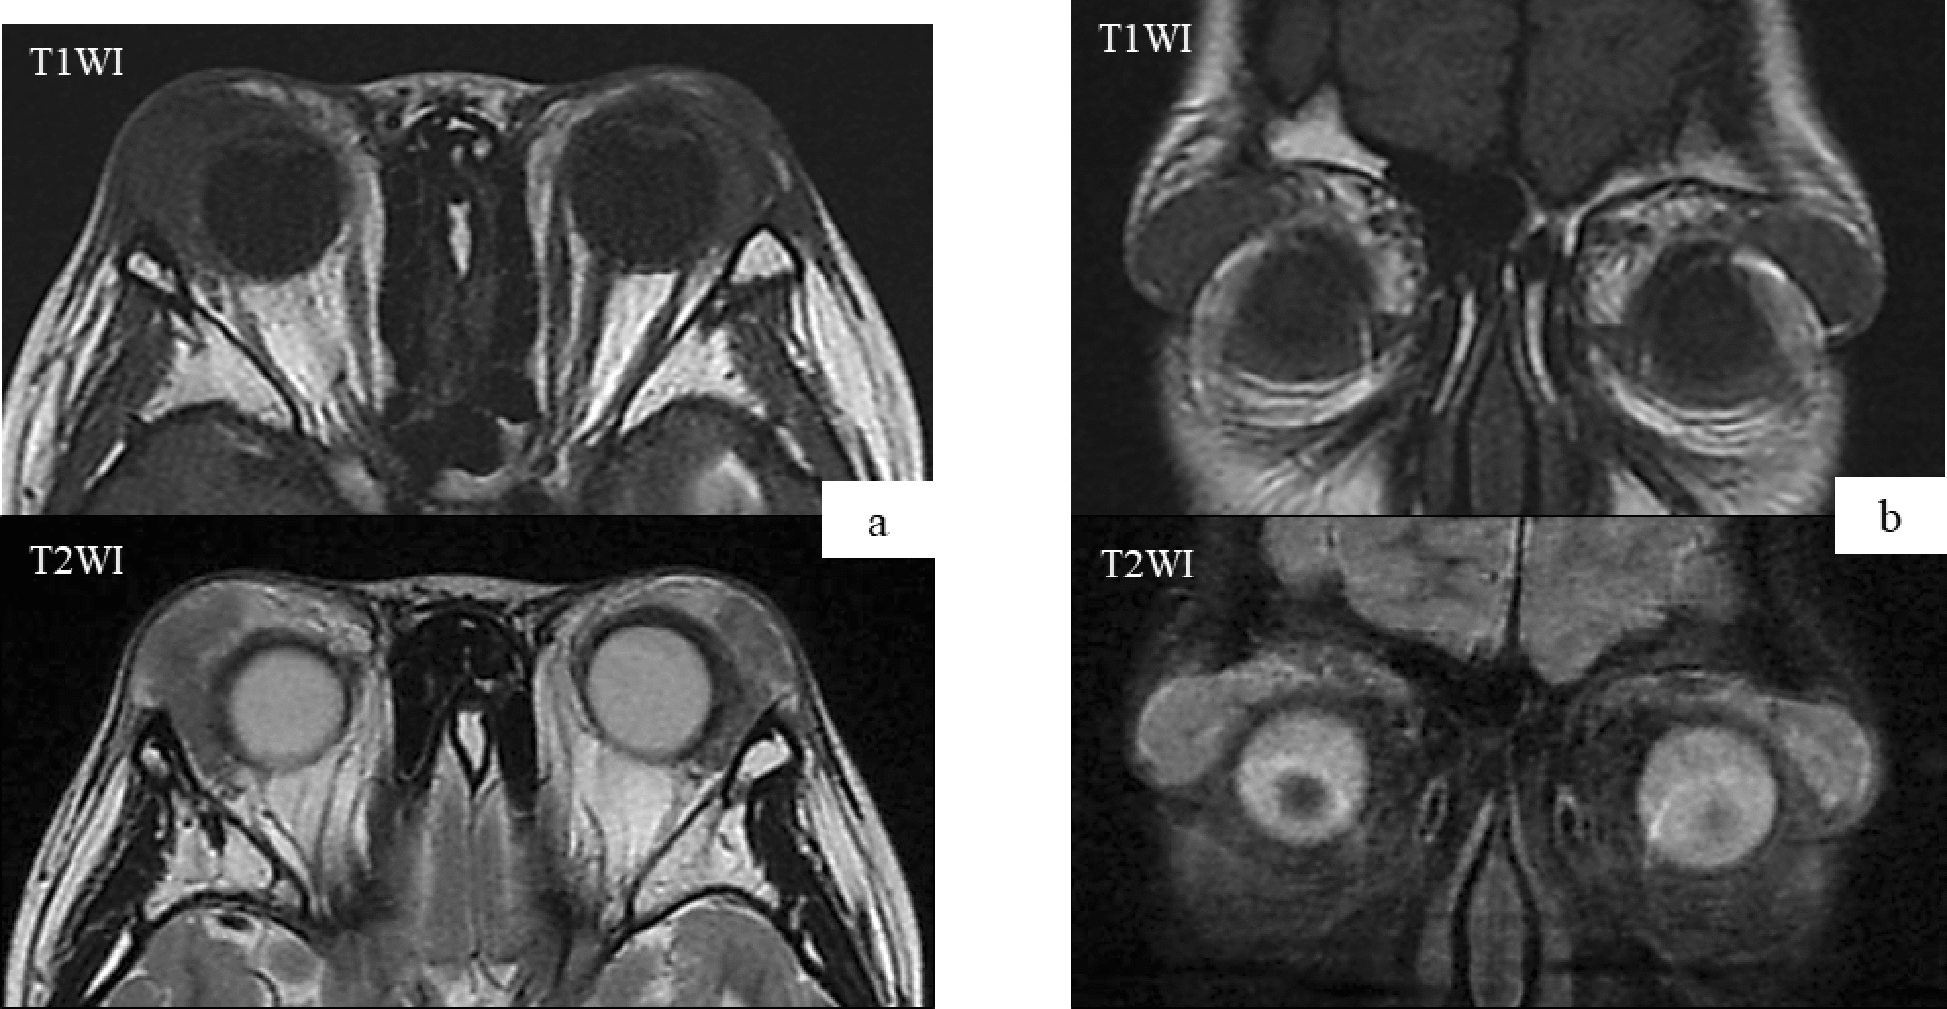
Magnetic resonance imaging. a, b T2-weighted axial and coronal MRI demonstrated the presence of an enhanced lesion of the bilateral lacrimal gland
